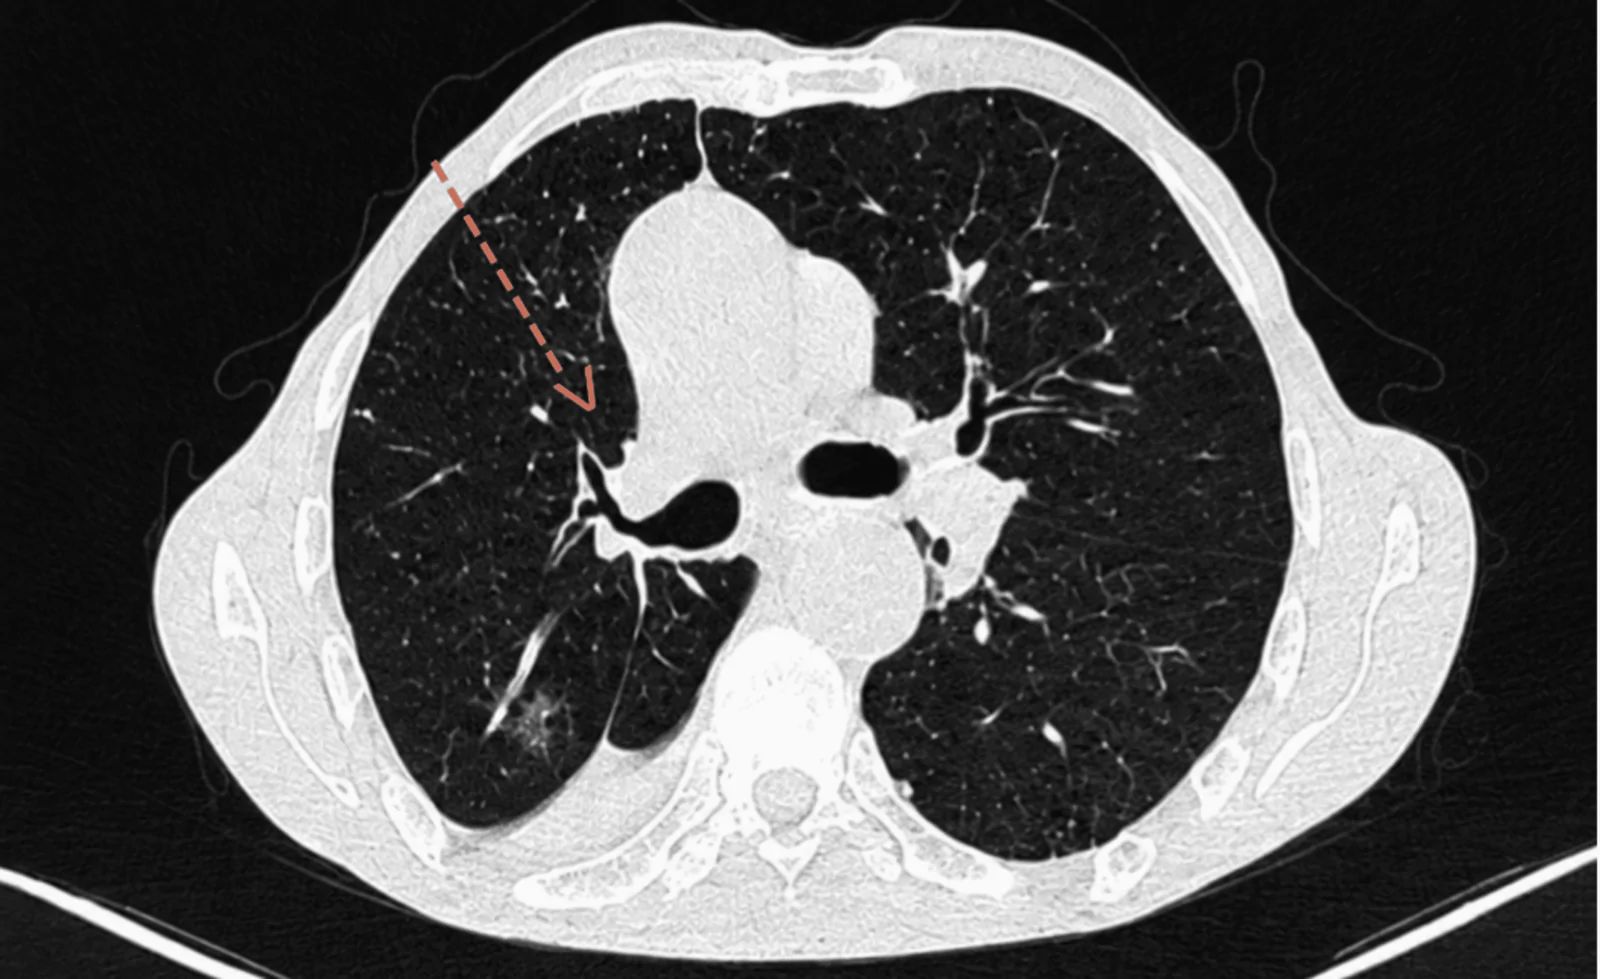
Contrast-enhanced chest computed tomography demonstrating a right hilar mass obstructing the bronchus intermedius
